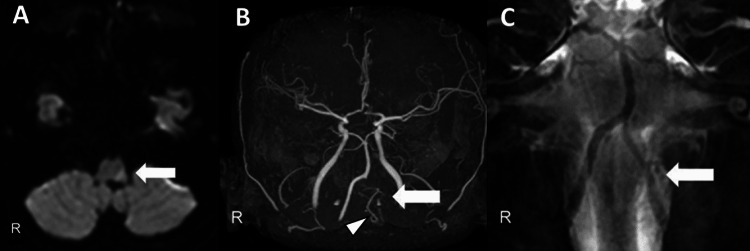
Magnetic resonance images upon hospitalization. (A) Axial image of diffusion-weighted imaging shows hyperintensity in the lateral medulla on the left side (white arrow). (B) Time-of-flight magnetic resonance angiography shows a poor depiction of the left vertebral artery (VA) at the middle segment of V4 (white arrow) and flow signal of the left posterior inferior cerebellar artery (PICA) (white arrowhead). (C) Basi-parallel anatomical scanning shows the V4 segment of the left VA and the bifurcation of the left PICA (white arrow).
